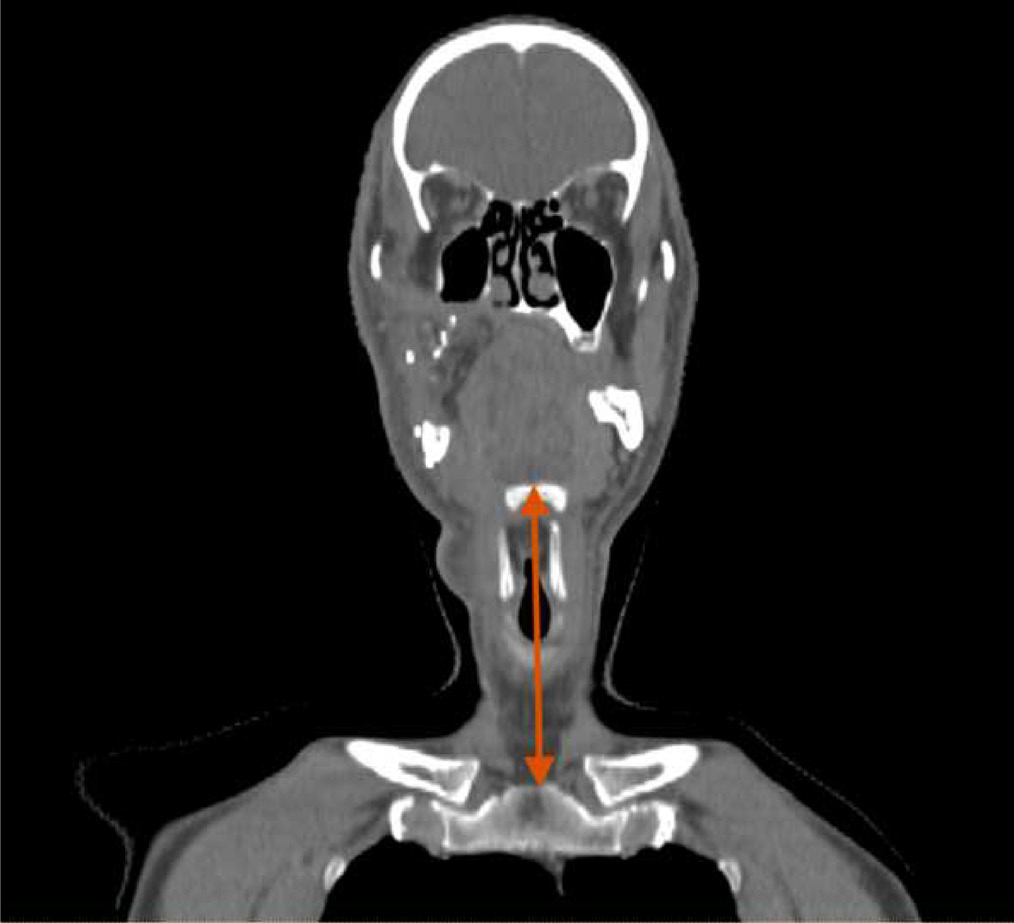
An example of neck length measurement on a coronal CT slice. Neck length was defined as the distance between the most cranial aspects of the hyoid bone and sternal notch (double ended arrow in red).
